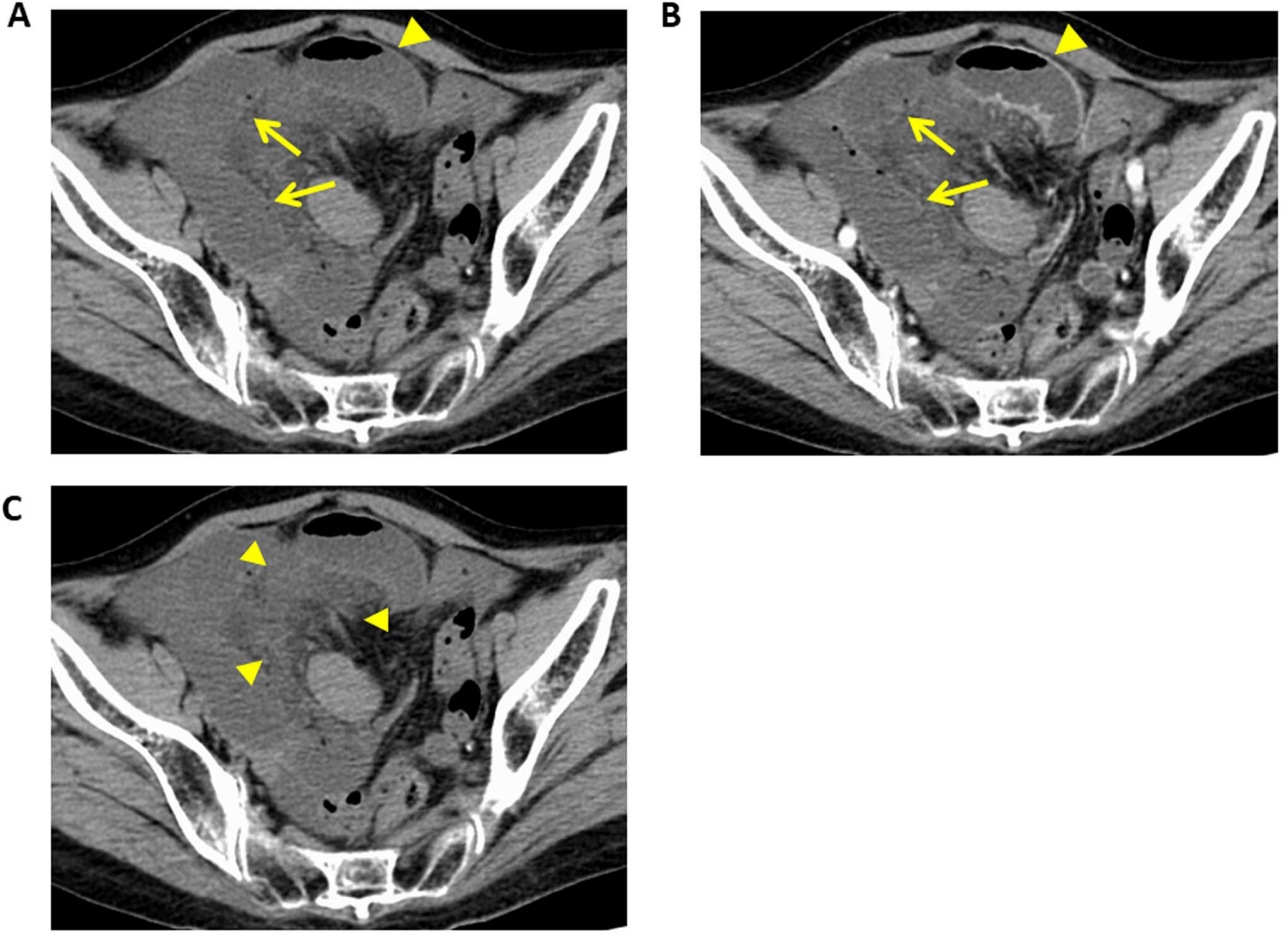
Strangulated small bowel obstruction due to an incarcerated hernia in a 69-year-old woman. A Unenhanced CT image, B Contrast-enhanced CT image, C Unenhanced CT image (same image as in 4A). A, B The CT images show that the involved (strangulated) small bowel segments (arrows) are not contrast enhanced while the uninvolved segments are contrast enhanced (arrow heads). C Mesenteric stranding (arrow heads) is seen
